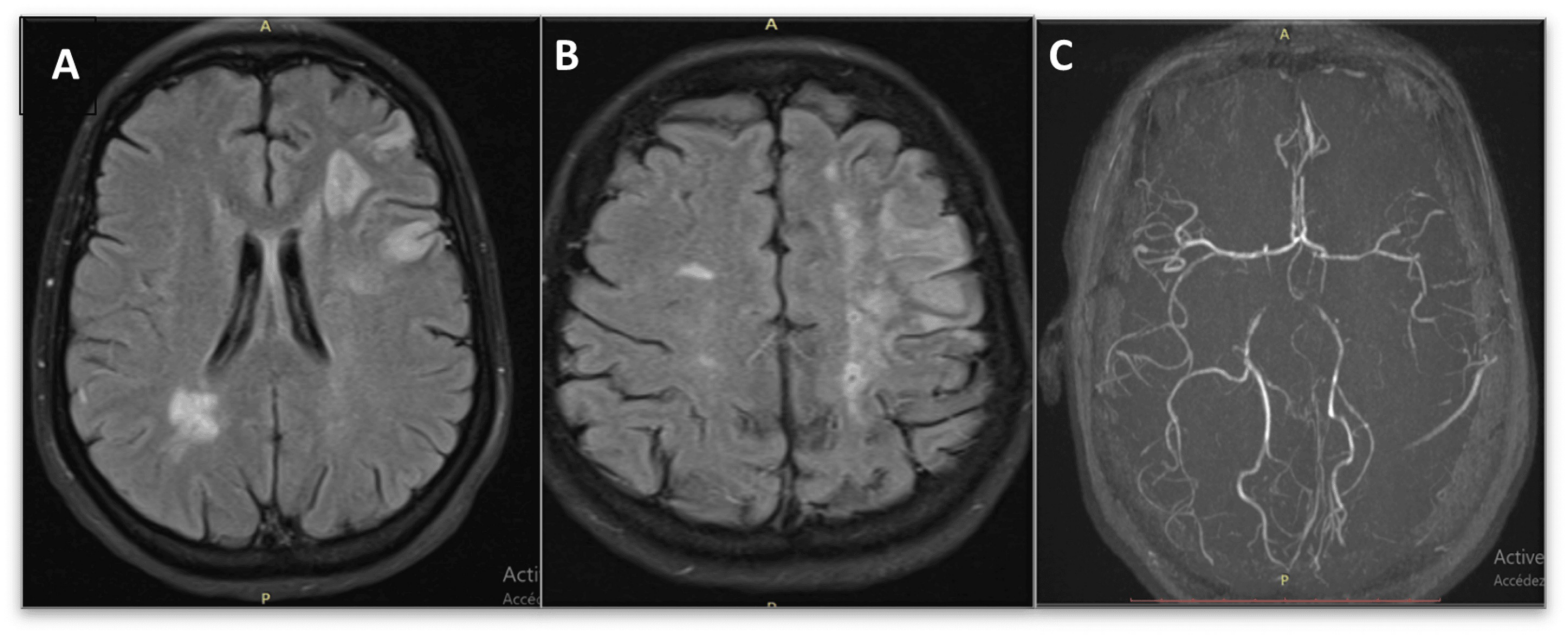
MRI with axial slices: (A, B) FLAIR sequence showing ischemic strokes of different ages. (C) MRA: irregular appearance of the intracranial arteries, particularly the left carotid artery MRI: magnetic resonance imaging, FLAIR: fluid-attenuated inversion recovery, MRA: magnetic resonance angiography
